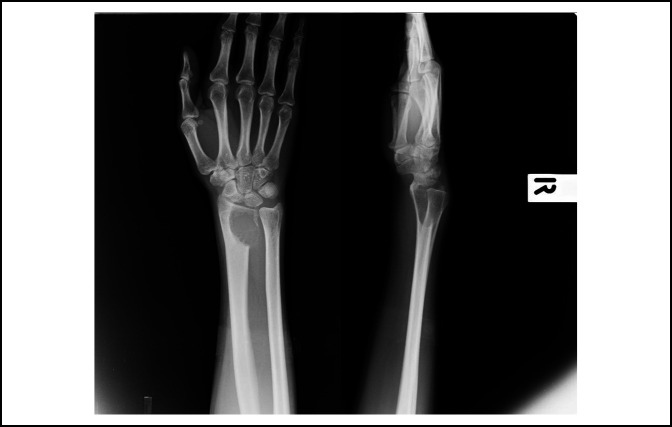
Radiographs demonstrating an osteolytic lesion with ill-defined margin of the distal radius on PA and lateral views.
